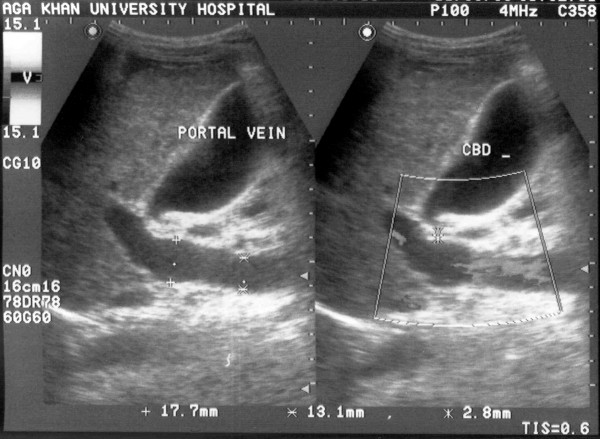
Ultrasound upper abdomen showing portal vein of 17.7 mm, coarse liver parenchyma.
